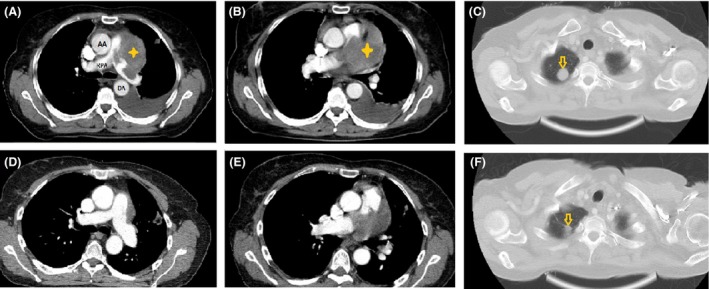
Intravenous contrast‐enhanced CT scan images of patient's thorax. First row images are taken at first presentation and second row images are related to the last CT scan after 9 months of chemotherapy. (A, B) (in mediastinal window) show a left mediastinal mass (which invades the main pulmonary trunk and left main pulmonary artery. After treatment, CT scan (D, E) showed more than 30% size reduction of the mass, suggestive of partial response. (C) (lung window) shows a metastatic nodule (yellow arrow) in pulmonary left upper lobe which becomes faint and smaller (F) after treatment. AA, ascending aorta; RPA, right pulmonary artery; DA, descending aorta.
